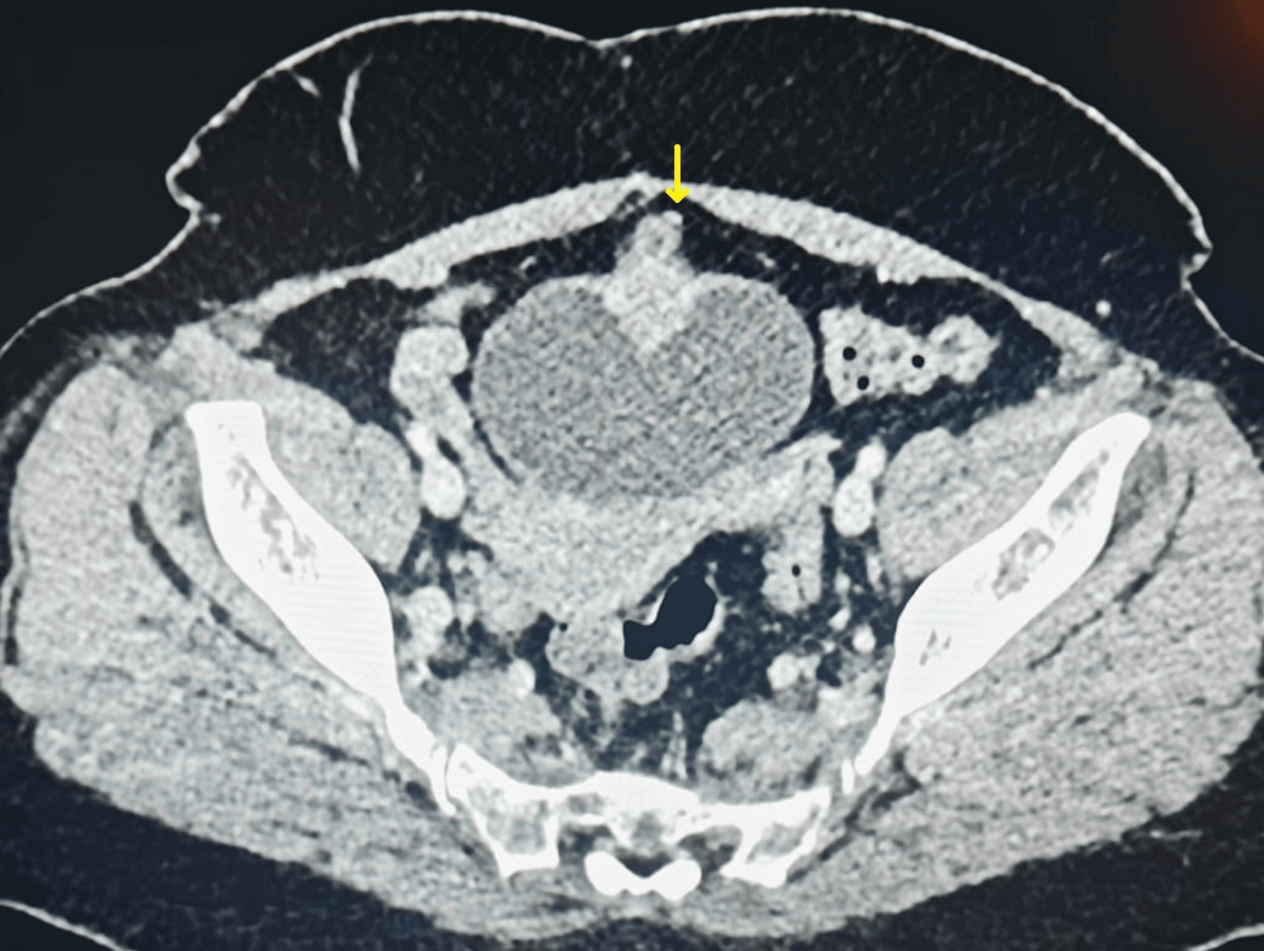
CT axial section showing extravesical extension into urachal remnants Yellow arrow pointing toward the extravesical extension CT: computed tomography
